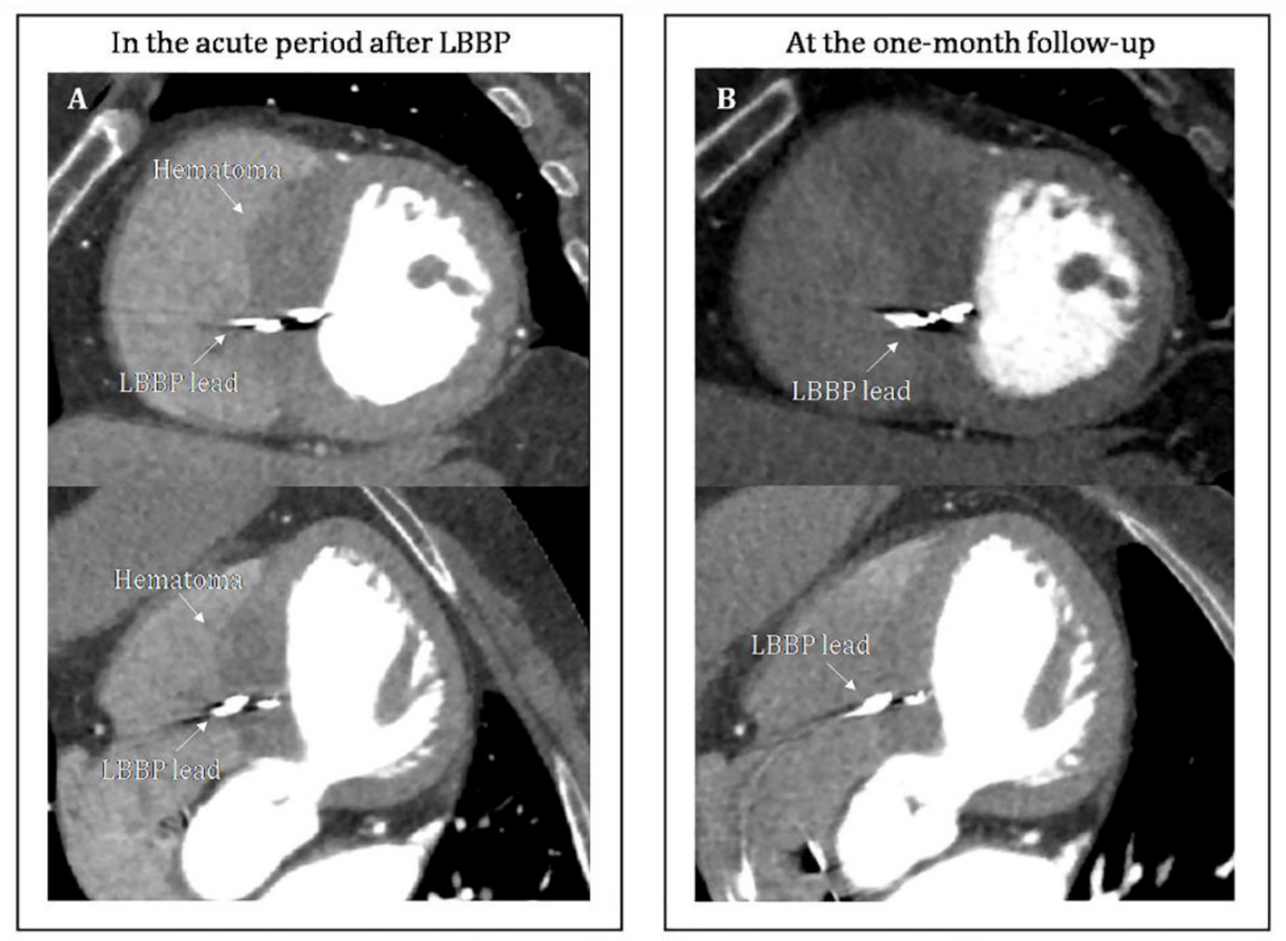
(A) CTA showed a hematoma in the basal segment of the interventricular septum in the acute period after LBBP. On non-contrast CTA, a high-density thickening in the interventricular septum was found. Post contrast, no significant change in the enhancement was observed. (B) CTA confirmed the hematoma has resolved at the 1-month follow-up. CTA, computed tomography angiography.
